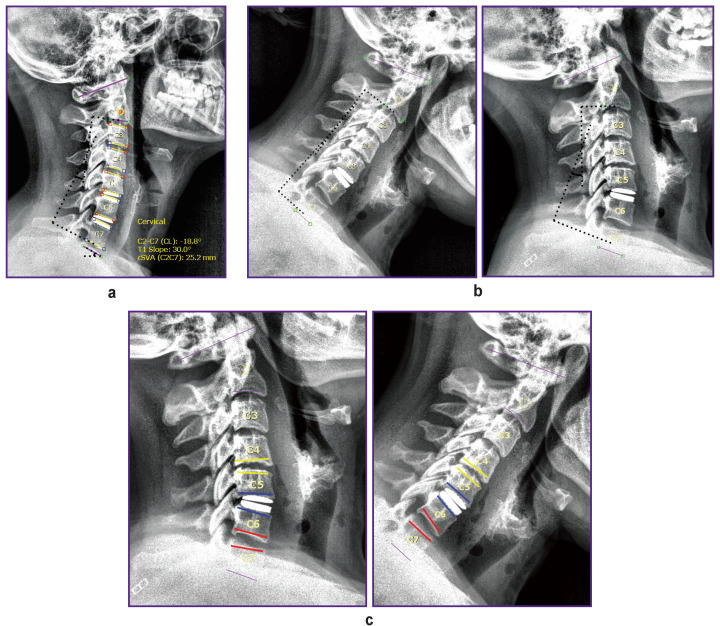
Radiography of the cervical spine and measurement of its parameters using Surgimap V2.2 software: (a) marking of CL, T1 slope, and cSVA parameters in static lateral projection; (b) CL marking in lateral projection to determine cervical spine mobility after arthroplasty at flexion and extension; (c) motion range determination of cervical spine segments after arthroplasty by measuring interbody space angle
